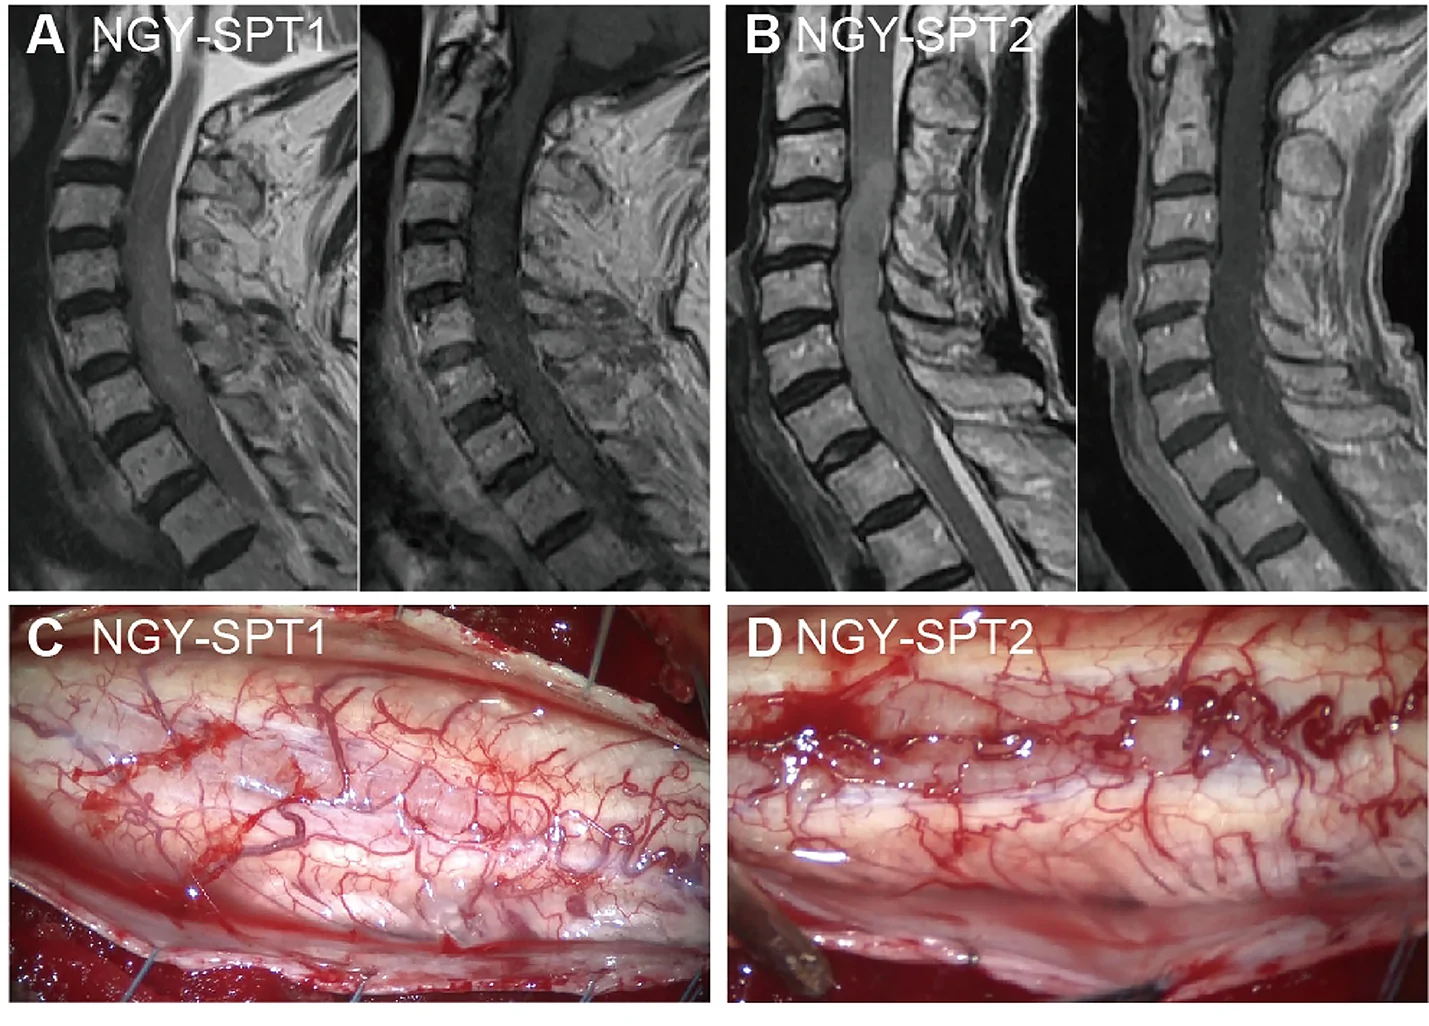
Preoperative magnetic resonance imaging (MRI) and intraoperative findings. (A and B) MRI of the two cases. T2-weighted images (left panels) show lesions that are hyperintense relative to the surrounding spinal cord parenchyma in both cases. T1 gadolinium‐enhanced images (right panels) demonstrate that the tumors are isointense to hypointense, with partial contrast enhancement observed in case NGY-SPT2. (C and D) Intraoperative findings of the spinal cord surface of two cases (NGY-SPT1 and 2). Arrows point to the part of the tumor exposed extramedullary
